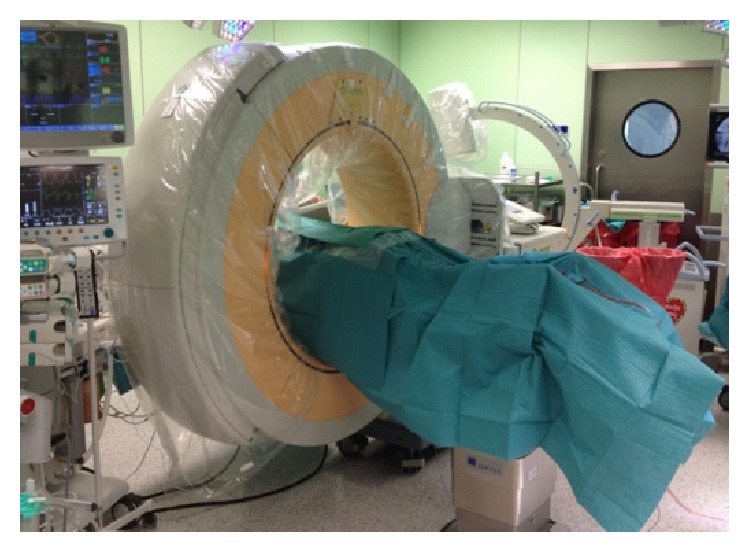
Intraoperative computed tomography evaluation with O-Arm (Medtronic).
